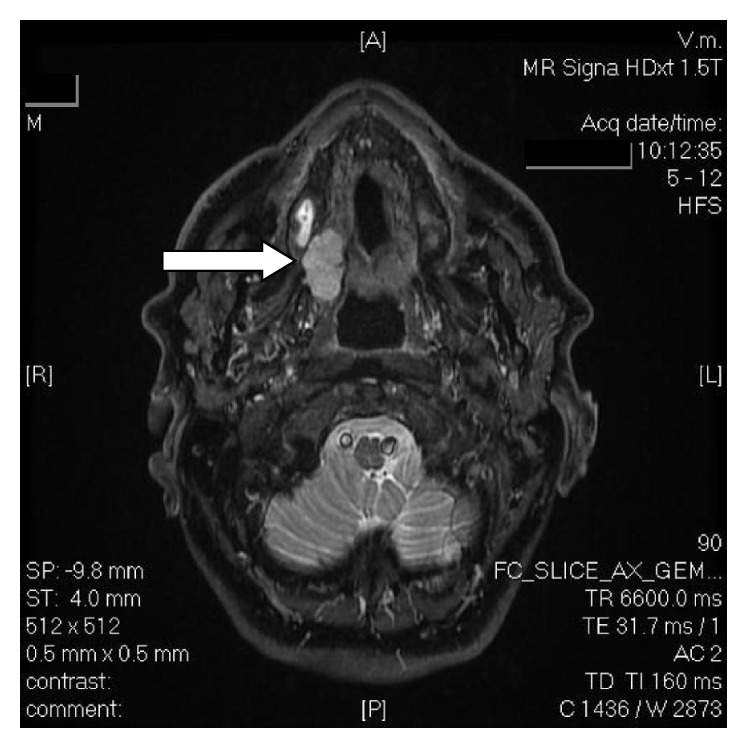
Preoperative horizontal MRI scan indicating the location (white arrow) of the adenocarcinoma of the right palatomaxillary region. [L]: left side; [R]: right side; [A]: anterior aspect; [P]: posterior aspect.
